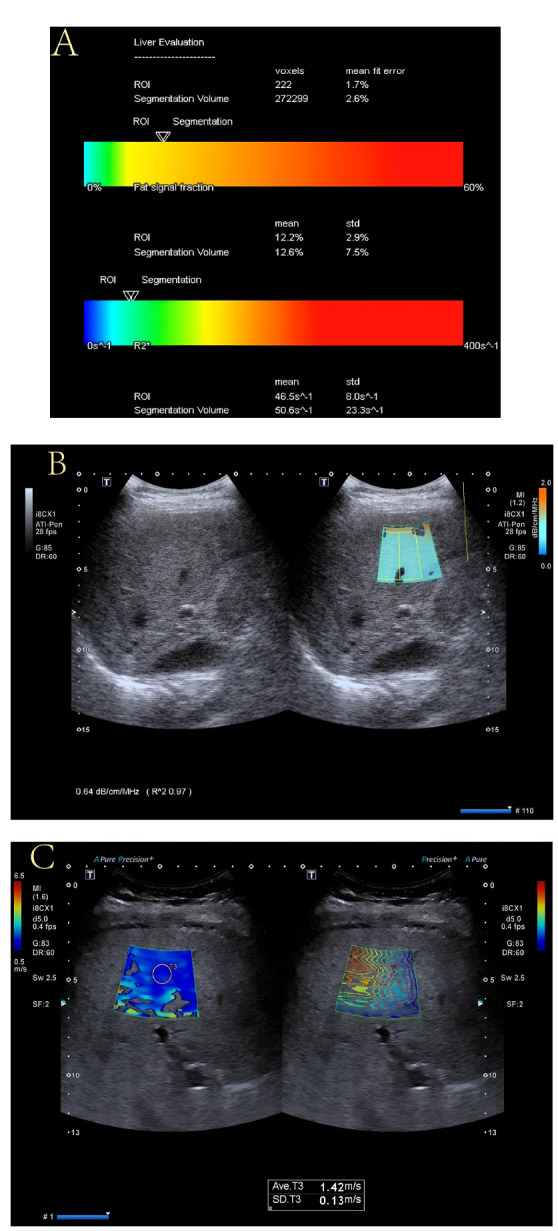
ATI and SWE maps of mild fatty liver. A: FF value was 12.2%, suggesting the patient to have a mild fatty liver. B. On the measurement of ultrasound attenuation imaging, the ATI was 0.79 dB/cm/MHz. C. On the measurement of elastic shear wave imaging, the SWE was 1.42 m/s.
